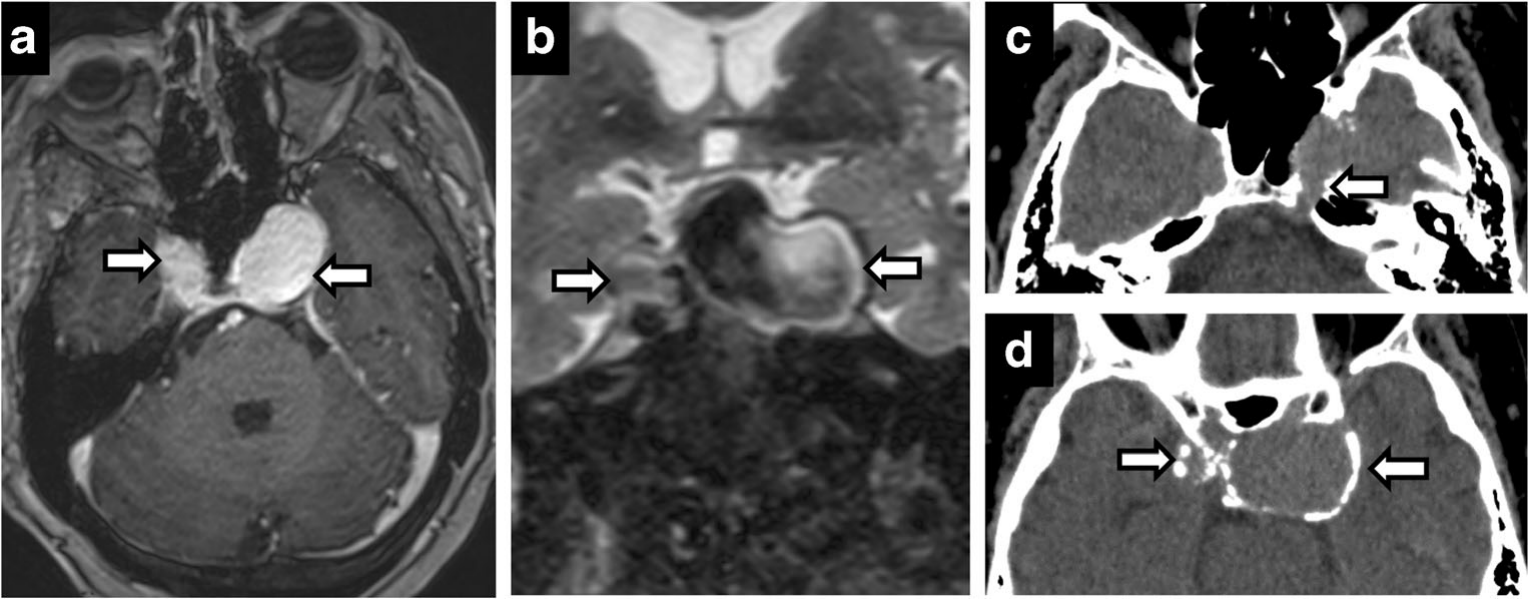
Bilateral cavernous ICA aneurysms. Axial C+ (a), coronal T2 (b), CT (c) and (d). A 73-year-old woman with left facial pain. Bilateral cavernous enhancing, partially calcified lesions encroaching the Meckel’s cave—left greater than right
